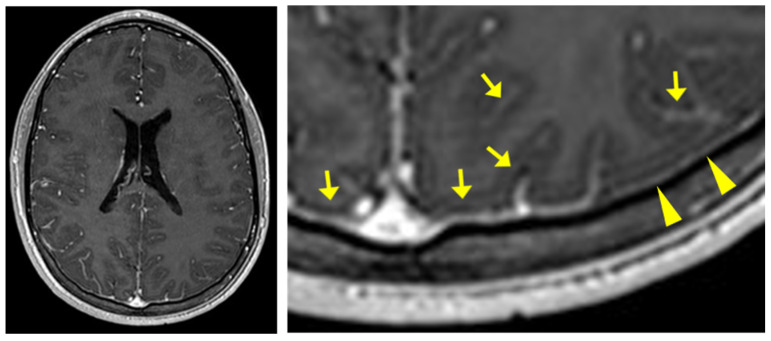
cMRI with a gadobutrol-enhanced T1-weighted fastfield echo (T1-FFE) sequence performed three days after lumbar puncture showing slight pachy- (arrowheads) and leptomeningeal (arrows) enhancement.
